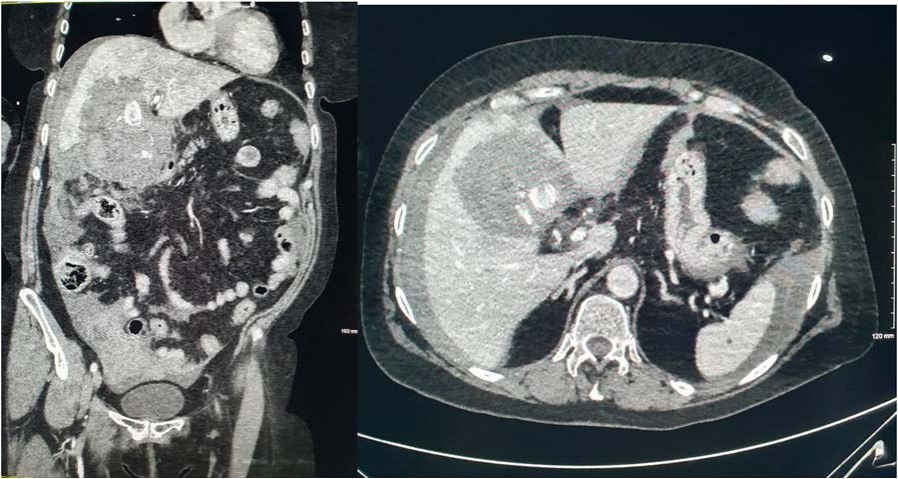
CT abdomen and pelvis with intravenous contrast in the portal venous phase was performed demonstrating perforated acute calculous cholecystitis and active haemorrhage.
